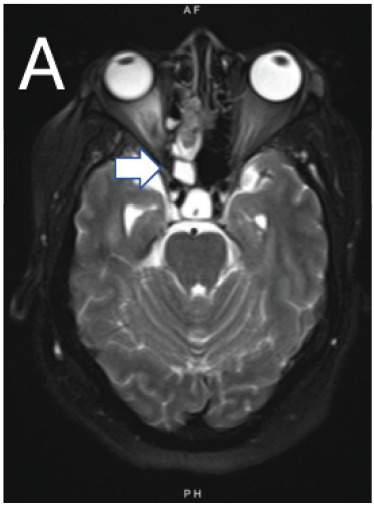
Axial T2-weighted magnetic resonance imaging of the brain demonstrating herniation of the right inferior frontal lobe tissue consistent with primary encephalocele (arrow).
